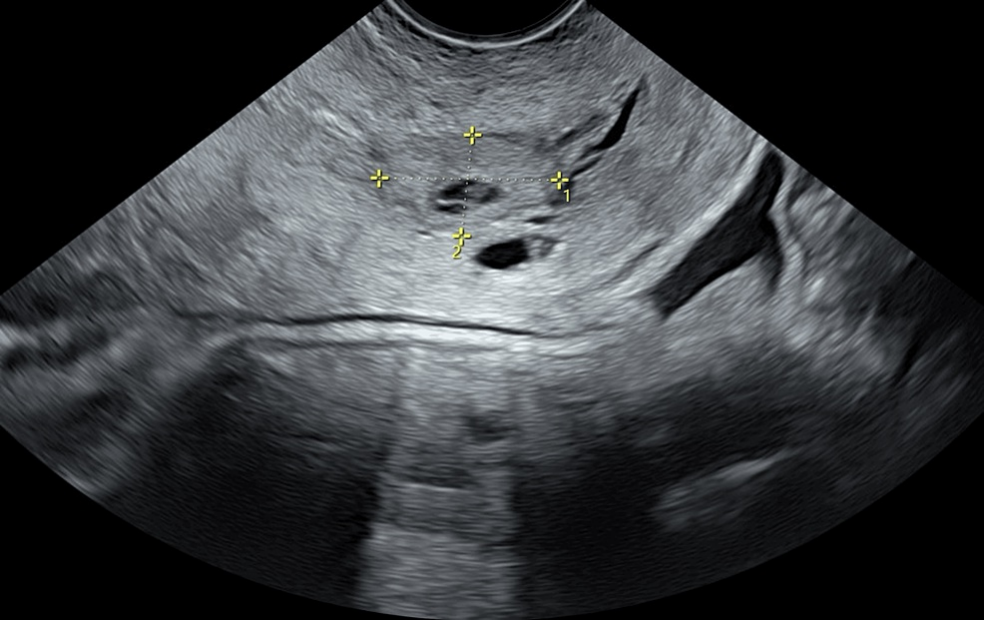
Transvaginal ultrasonography after KCl injection revealed that a hypervascular echogenic change was shown in the cervical pregnancy
